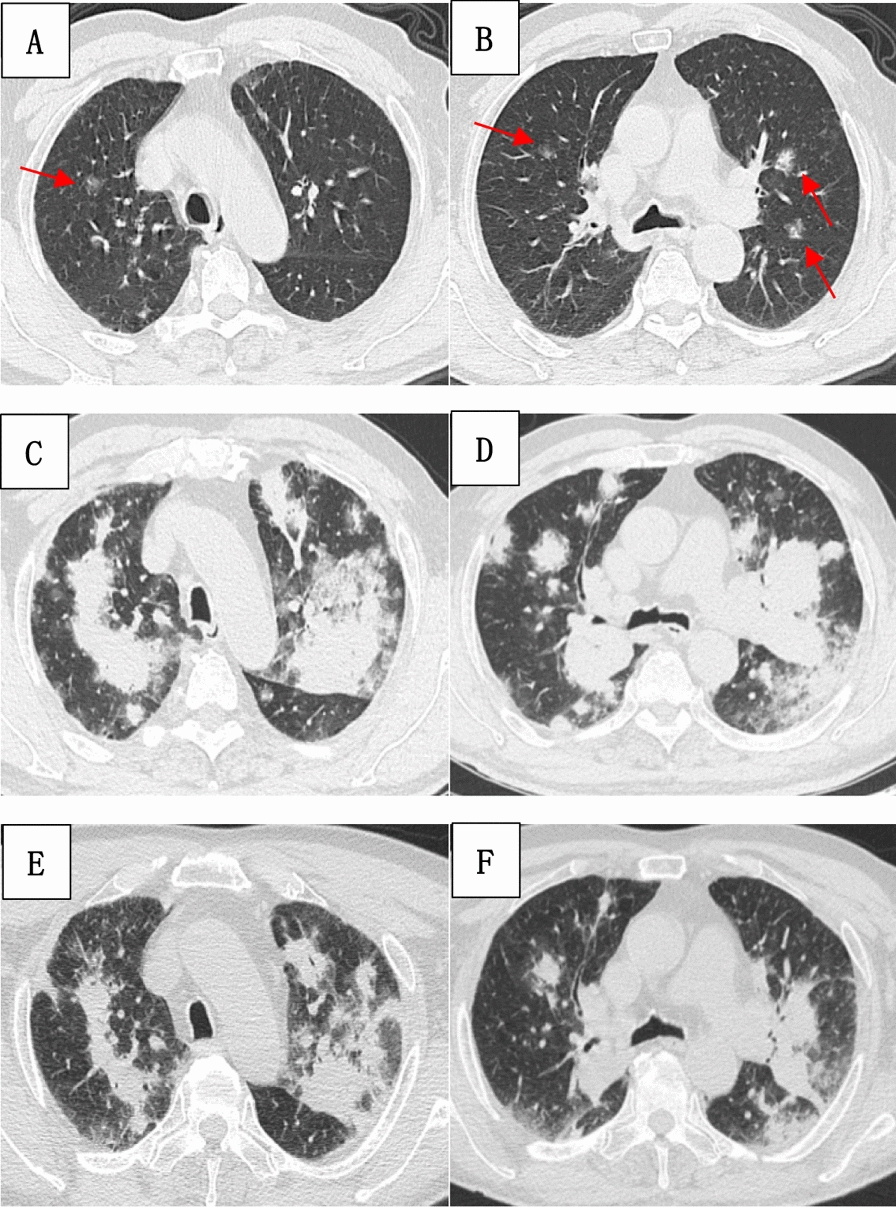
Chest computed tomography scan findings demonstrating the progression of lung lesions over time. (A, B) Initial presentation on 16 October 2023, with multiple small nodular shadows in both lungs (red arrows). (C, D) Progression to multiple consolidations and nodular high-density shadows on 21 December 2023. (E, F) Partial resolution of consolidations and nodular shadows on 20 January 2024
